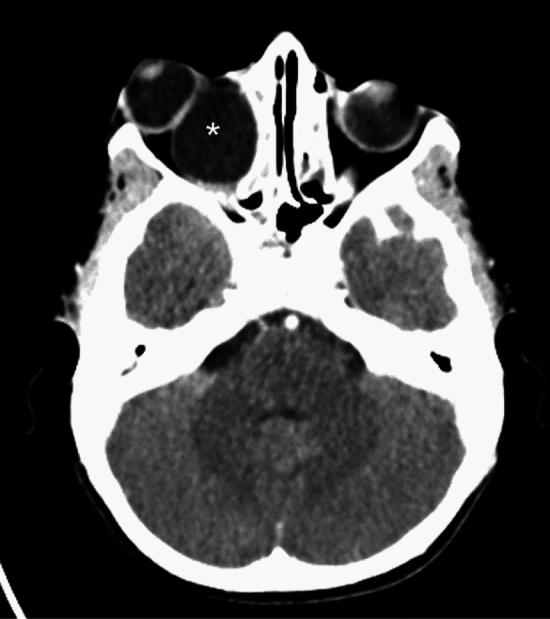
Computed tomography scan of the head, performed prior to surgery. The asterisk indicates the well-defined hydatid cyst in the right orbit
